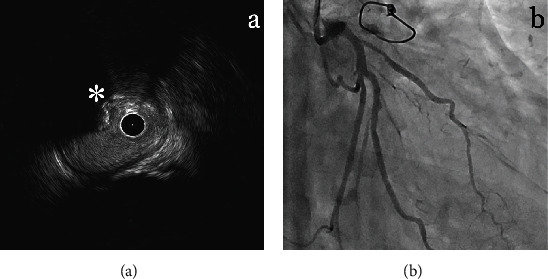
Intravascular imaging and final coronary angiography. Intravascular ultrasonography after rotational atherectomy revealed the presence of a calcified nodule (asterisk) (a). Final coronary angiography revealed TIMI3 flow to the left circumflex coronary artery after deployment of a drug-eluting stent.
